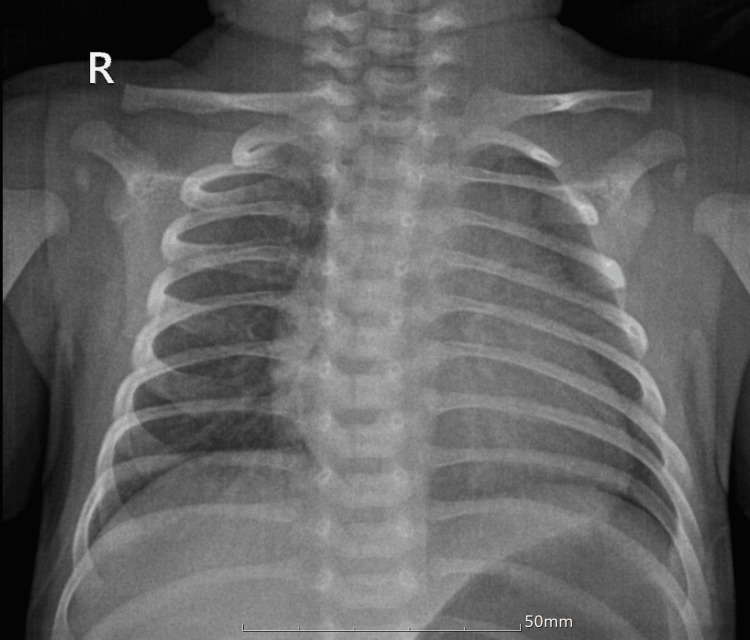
Chest radiography demonstrated bilateral lower lung field consolidations. The cardiac silhouette is within normal limits, and the bilateral costophrenic angles are preserved without evidence of pleural effusion.
